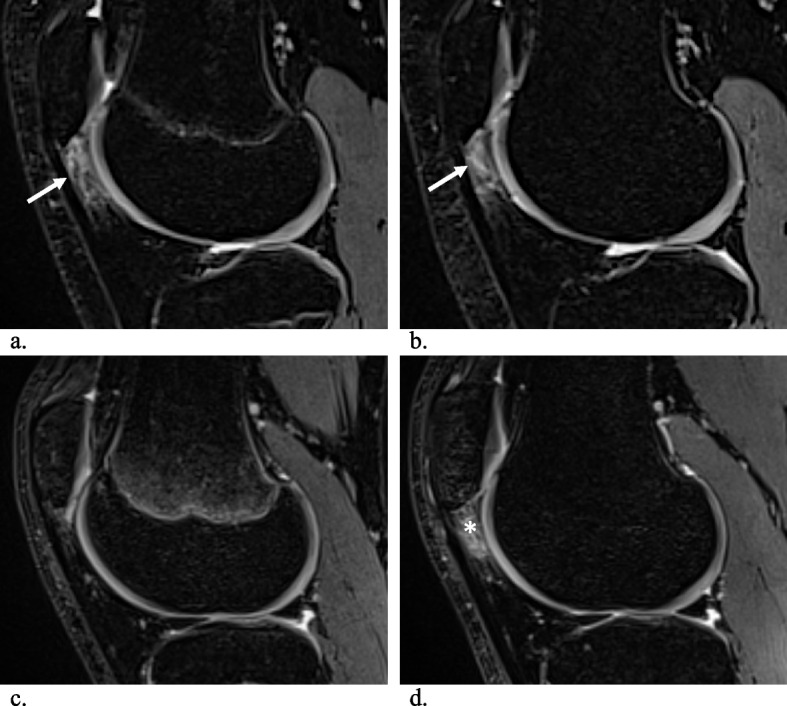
Sagittal MR images of a competitive youth alpine skier (a and b) showing persistent superolateral Hoffa fat pad edema (SHFP, arrows) of the right knee over a period of 48 months. A new SHFP edema of the left knee at the 48-month follow-up compared to baseline (asterisk) is shown at c and d
